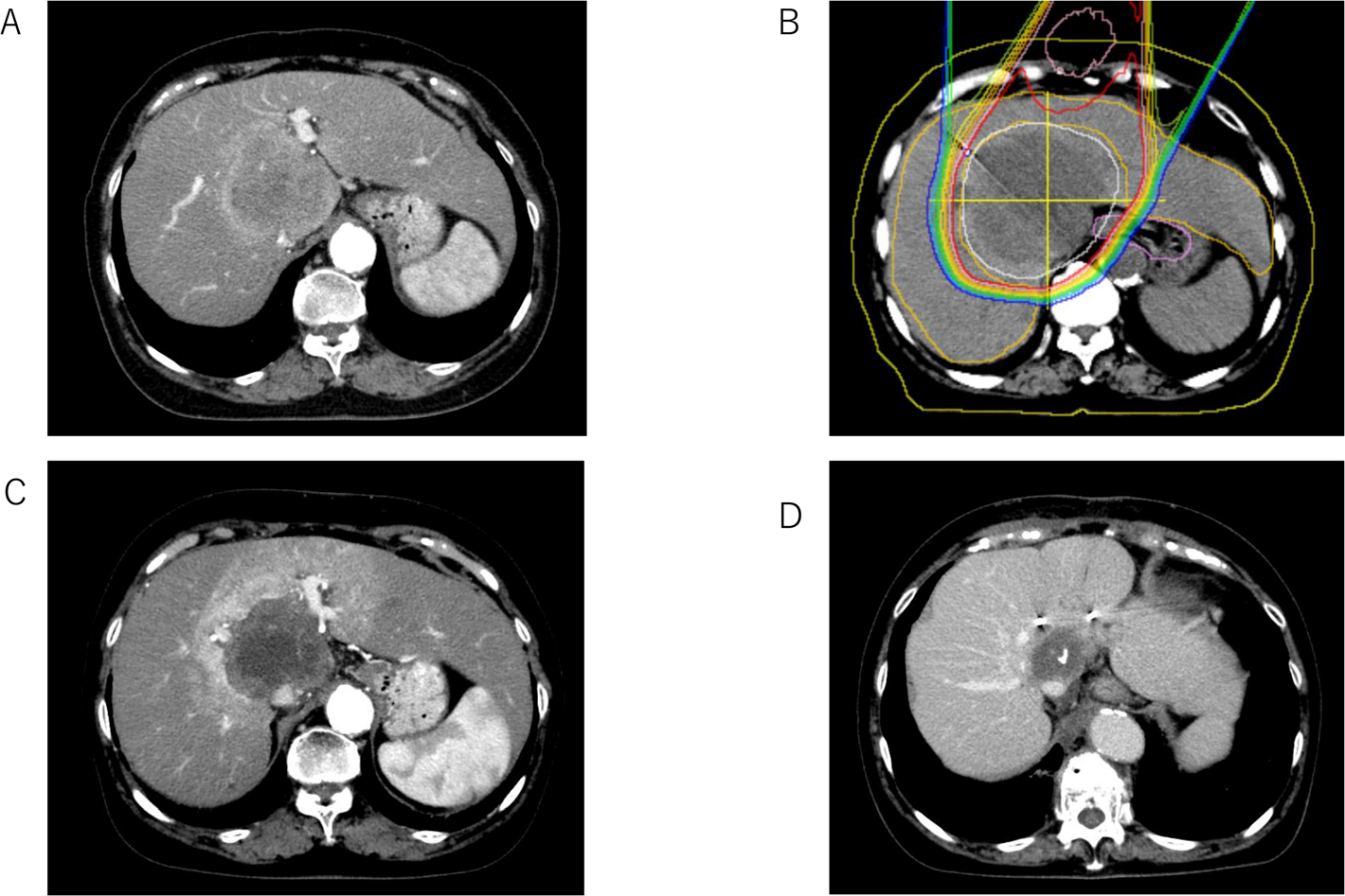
Imaging features of a 75-year-old man with a single intrahepatic 70 mm tumor at the caudate lobe who was diagnosed with intrahepatic cholangiocarcinoma. a Computed tomography at diagnosis. b The proton beam therapy dose line. c Six months after proton beam therapy. d Three years after proton beam therapy
